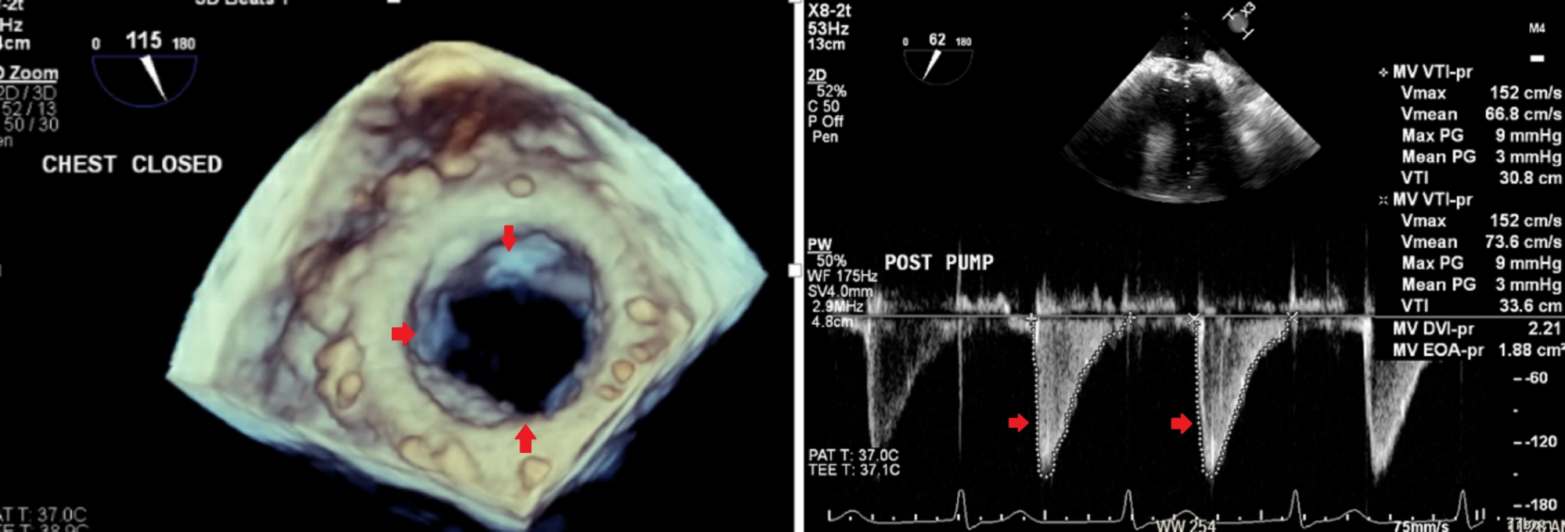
Transesophageal echocardiogram (three-dimensional view) after surgical mitral valve replacement showing normally function valve with normal gradients
